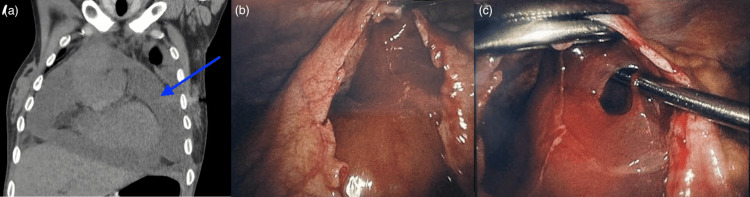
CT scan demonstrating a moderate-to-large pericardial effusion and intraoperative views showing loculations and adhesions. (A) Coronal chest CT image with blue arrow indicating a large pericardial effusion. (B, C) Intraoperative views through the pericardial window demonstrating thick, loculated pericardial fluid and dense adhesions between the pericardium and myocardium.
